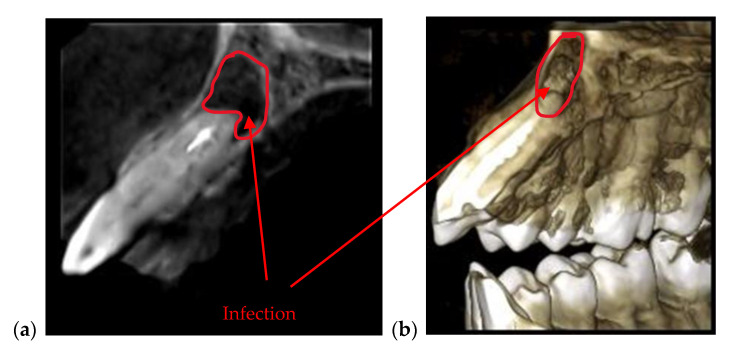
(a) Sagittal view and (b) 3D CBCT reconstruction of an infection formed at the tip of a tooth. Patient C.B.G., female, age 37 years, diagnosed with dental abscess.
